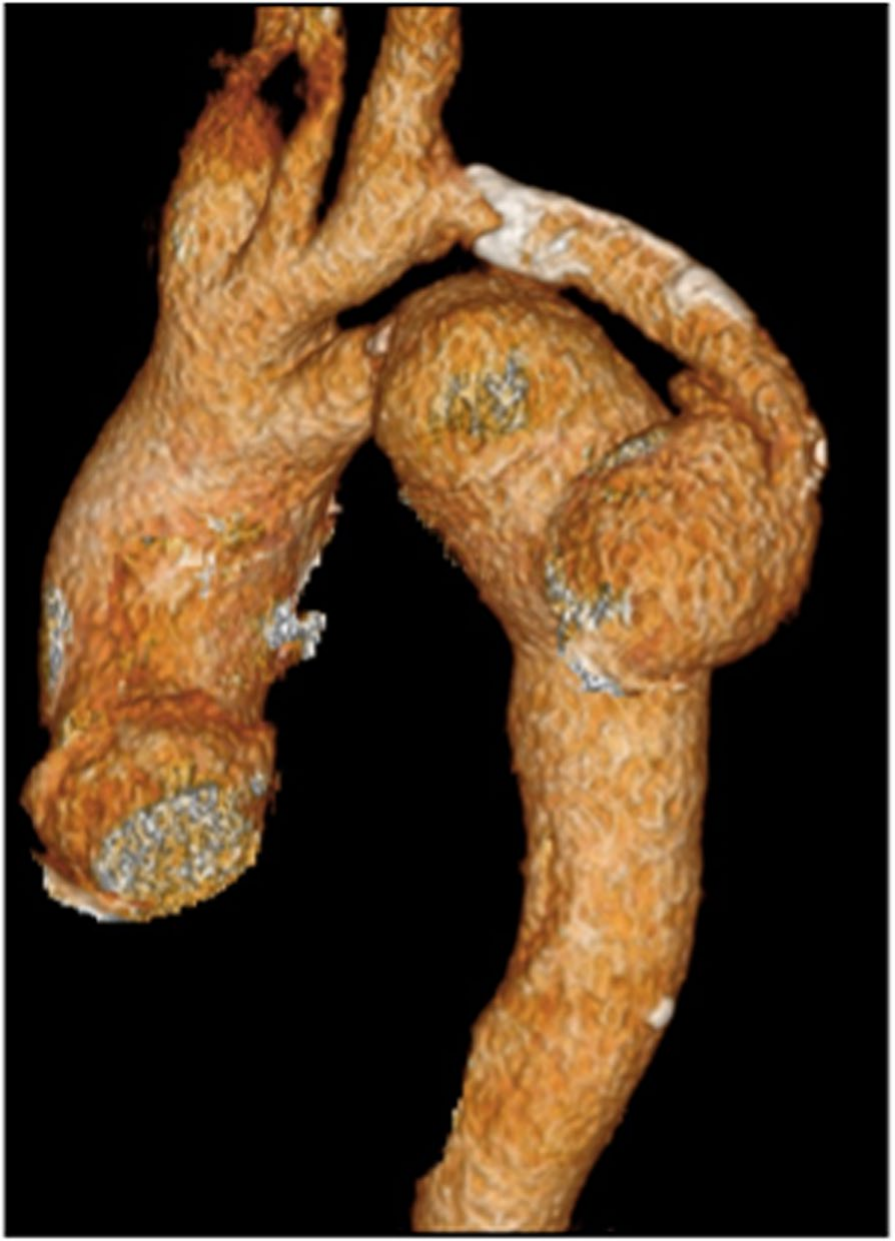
Three-dimensional computed tomography. Preoperative view. A pseudoaneurysm was formed at the distal anastomosis of the bypass graft
